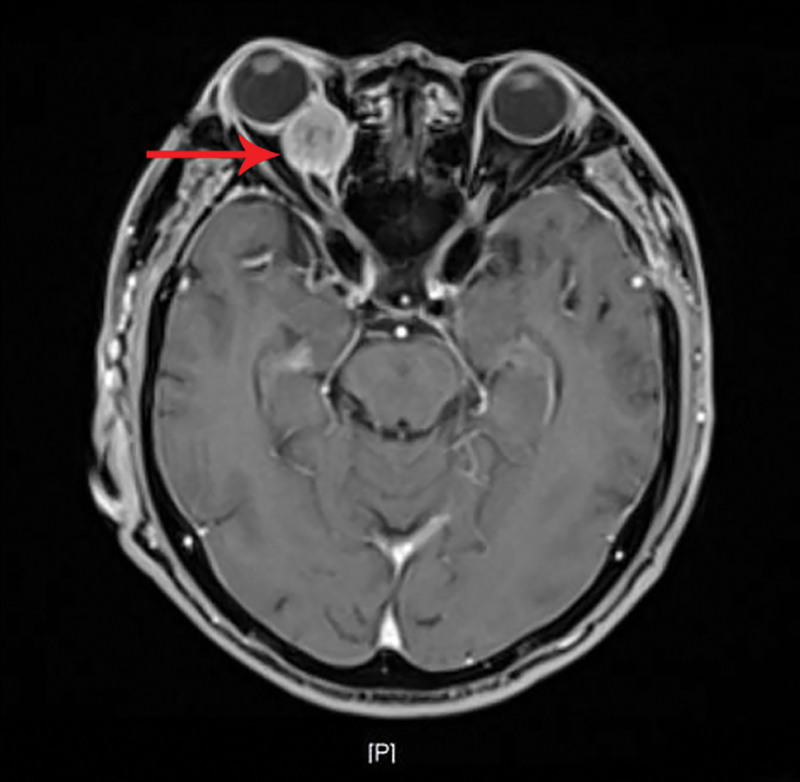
Contrast-enhanced magnetic resonance imaging showing a retrobulbar mass with a rapid wash-in and slow wash-out enhancement pattern (arrow).
